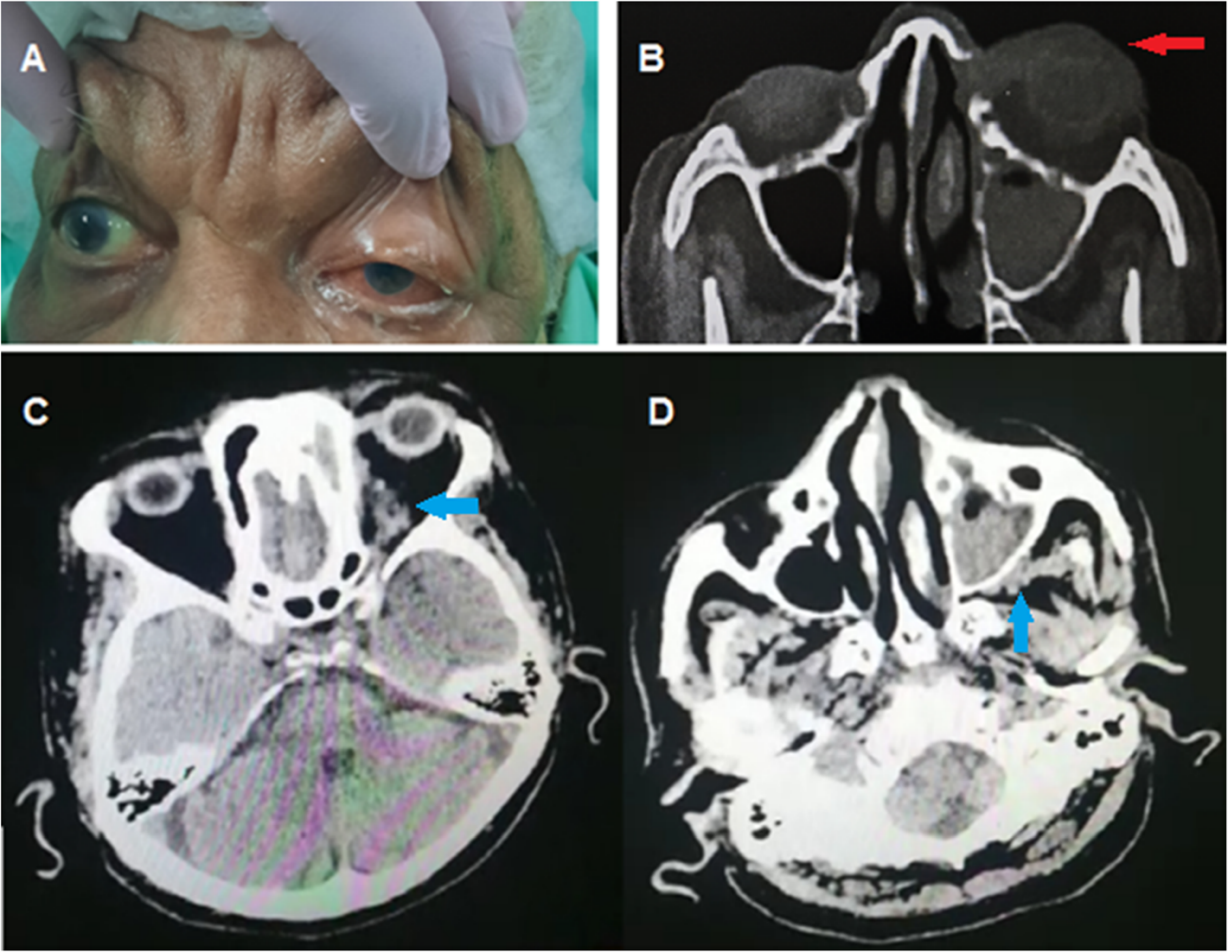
A Chemosis and total ophthalmoplegia of the left eye. B Red arrow showing proptosis of the left eye along paranasal CT. C Blue arrow pointing to infiltration of soft tissue retro orbit sharing in the presentation of orbital apex syndrome donating invasion from infected and inflamed sinuses. D Blue arrow representing infiltration of periantral fat donating invasion from nearby sinuses
